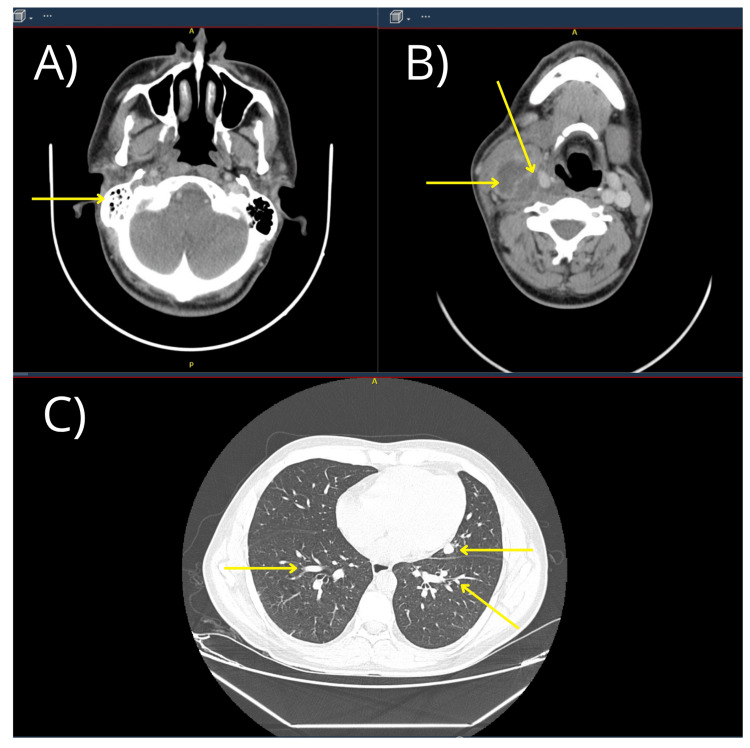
Contrast-enhanced computed tomography of the neck A) Mastoid level showing hypodensity in the right internal jugular vein compatible with venous thrombosis and right otomastoiditis. B) Cricoid cartilage level showing a collection in the right cervical region. C) Pulmonary level showing multiple bilateral peripheral nodular opacities, some demonstrating a probable feeding vessel sign, findings highly suggestive of septic emboli.
